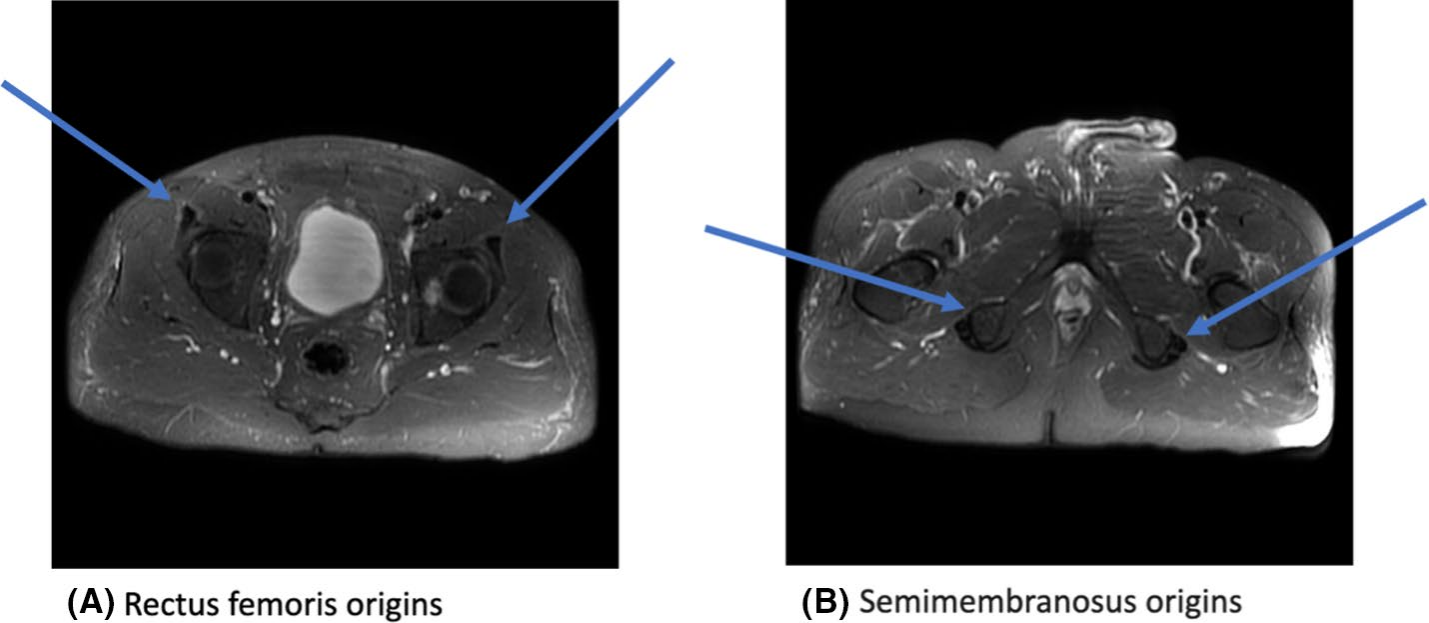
MRI pelvis with and without contrast one month after the initial MRI and a course of steroids showing complete resolution of the bilateral semimembranosus and left rectus femoris nonspecific edema and enhancement along with minimal residual right rectus femoris nonspecific edema and enhancement
